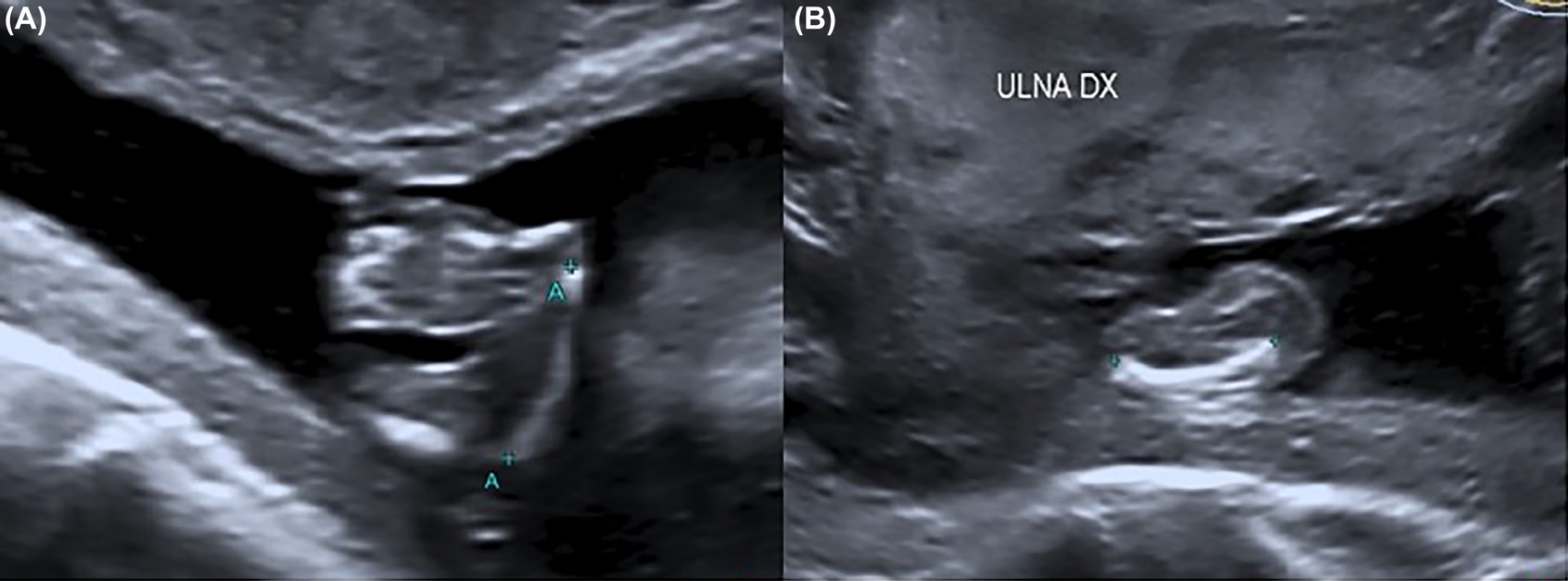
Ultrasonographic images of fetal upper limb defects at 18 weeks of gestation. (A) Twisted fetal hand. (B) Curved ulna and radio aplasia.
